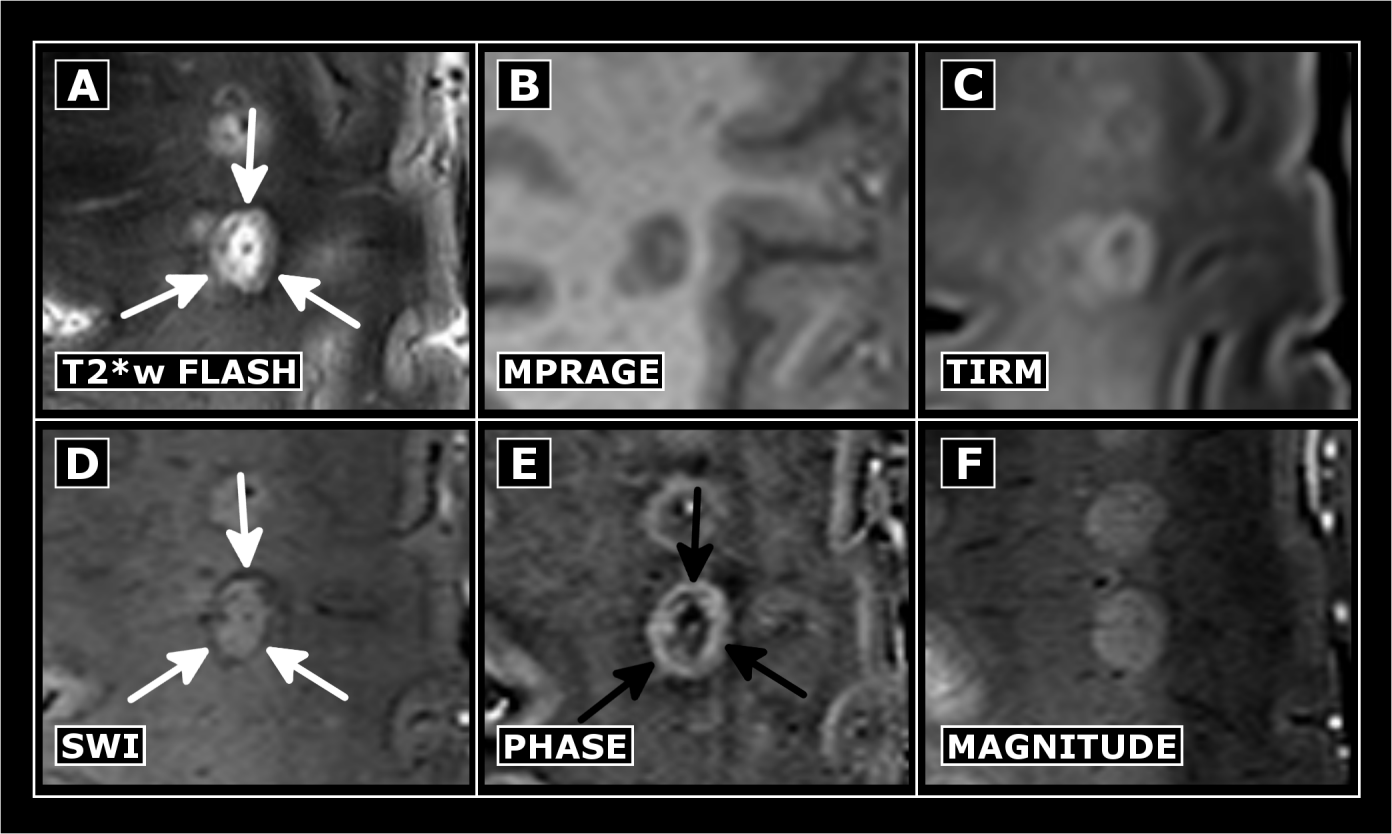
Exemplary chronic MS lesion. MS lesion in T2*w (A), T1w (B), FLAIR (C), SWI (D), phase (E) and magnitude (F) images. Prominent phase (black arrows) and corresponding T2*w/SWI hypointense rim are highlighted (white arrows).
